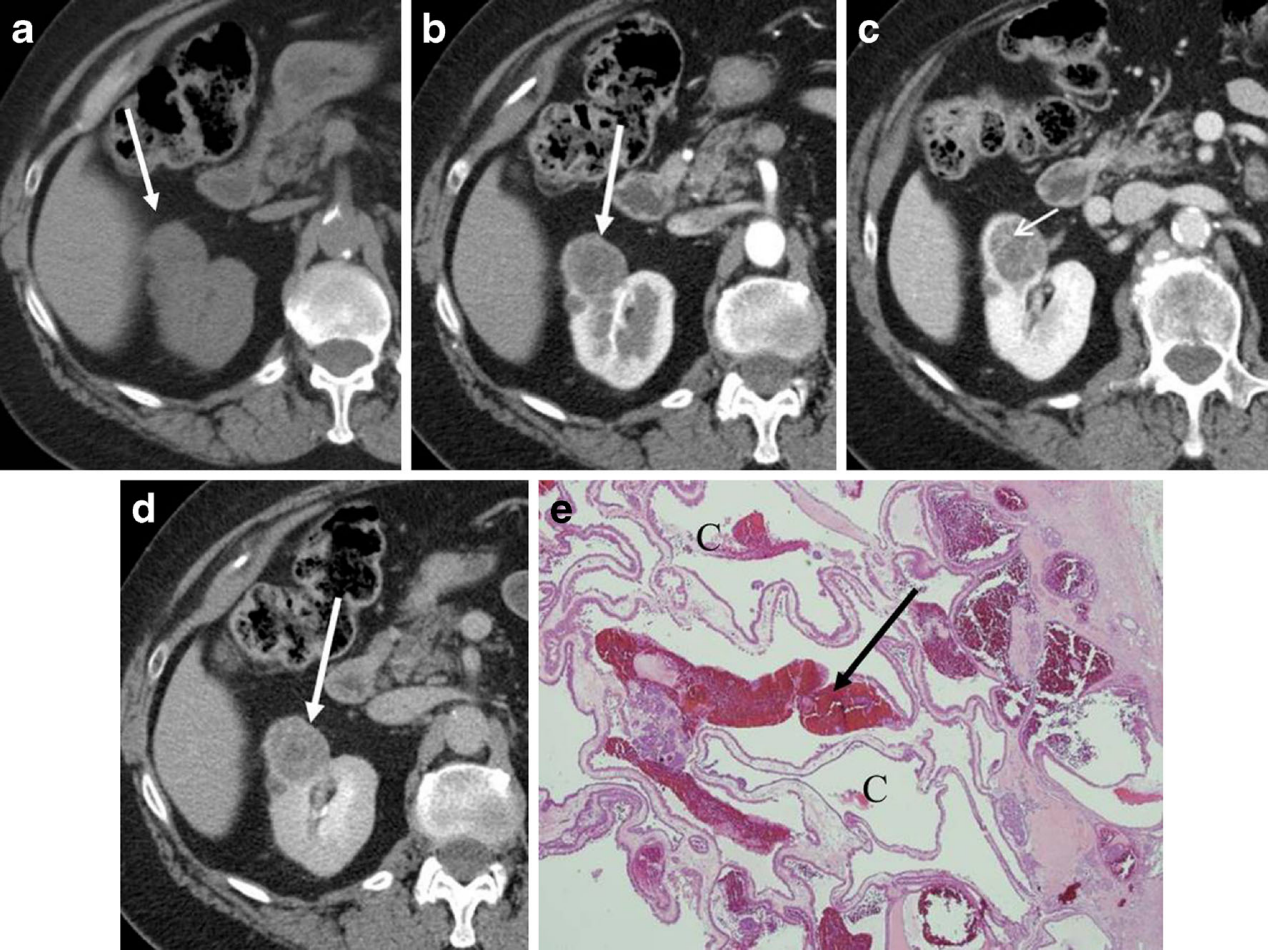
A 75-year-old woman with a right renal oncocytoma presenting as a hypovascular mass secondary to cystic change and haemorrhage. a Unenhanced axial CT image shows an exophytic right renal mass, which is iso-attenuating to the normal kidney (*arrow*). b The corticomedullary phase of the axial CE-CT shows the mass to be low attenuation (*arrow*). c The nephrographic phase demonstrates thin enhancing septations (*small arrow*). d The delayed phase shows the mass to be heterogeneous (*arrow*). e (Low magnification) Oncocytoma, cystic pattern, tumour cells predominantly arranged as cystic structures (*C*) with haemorrhage (*arrow*).
